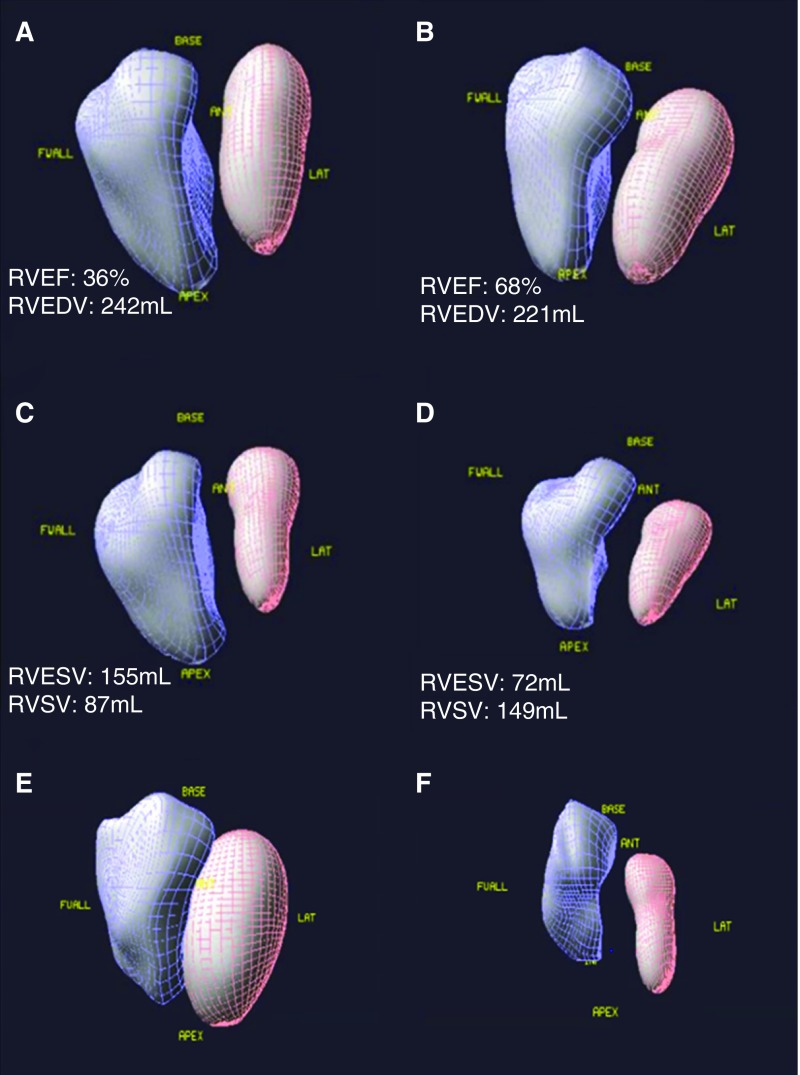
Ventricular images obtained by cadmium-zinc-telluride (CZT) single-photon emission computed tomography (SPECT) equilibrium radionuclide angiocardiography (ERNA). (*A*) The left (red) and right (blue) ventricles at end diastole in a treatment-naive participant. (*B*) The left and right ventricles at end diastole after 6 months of combination therapy with ambrisentan and tadalafil. (*C*) The left and right ventricles at end systole in the same treatment-naive participant. (*D*) The left and right ventricles at the end of systole after 6 months of combination therapy with ambrisentan and tadalafil. (*E*) CZT SPECT ERNA images excluded because of separation issues; the ventricles could not be resolved. (*F*) CZT SPECT ERNA images excluded because of low intensity (unable to be processed). RVEDV = right ventricular end-diastolic volume; RVEF = right ventricular ejection fraction; RVESV = right ventricular end-systolic volume; RVSV = right ventricular stroke volume.
